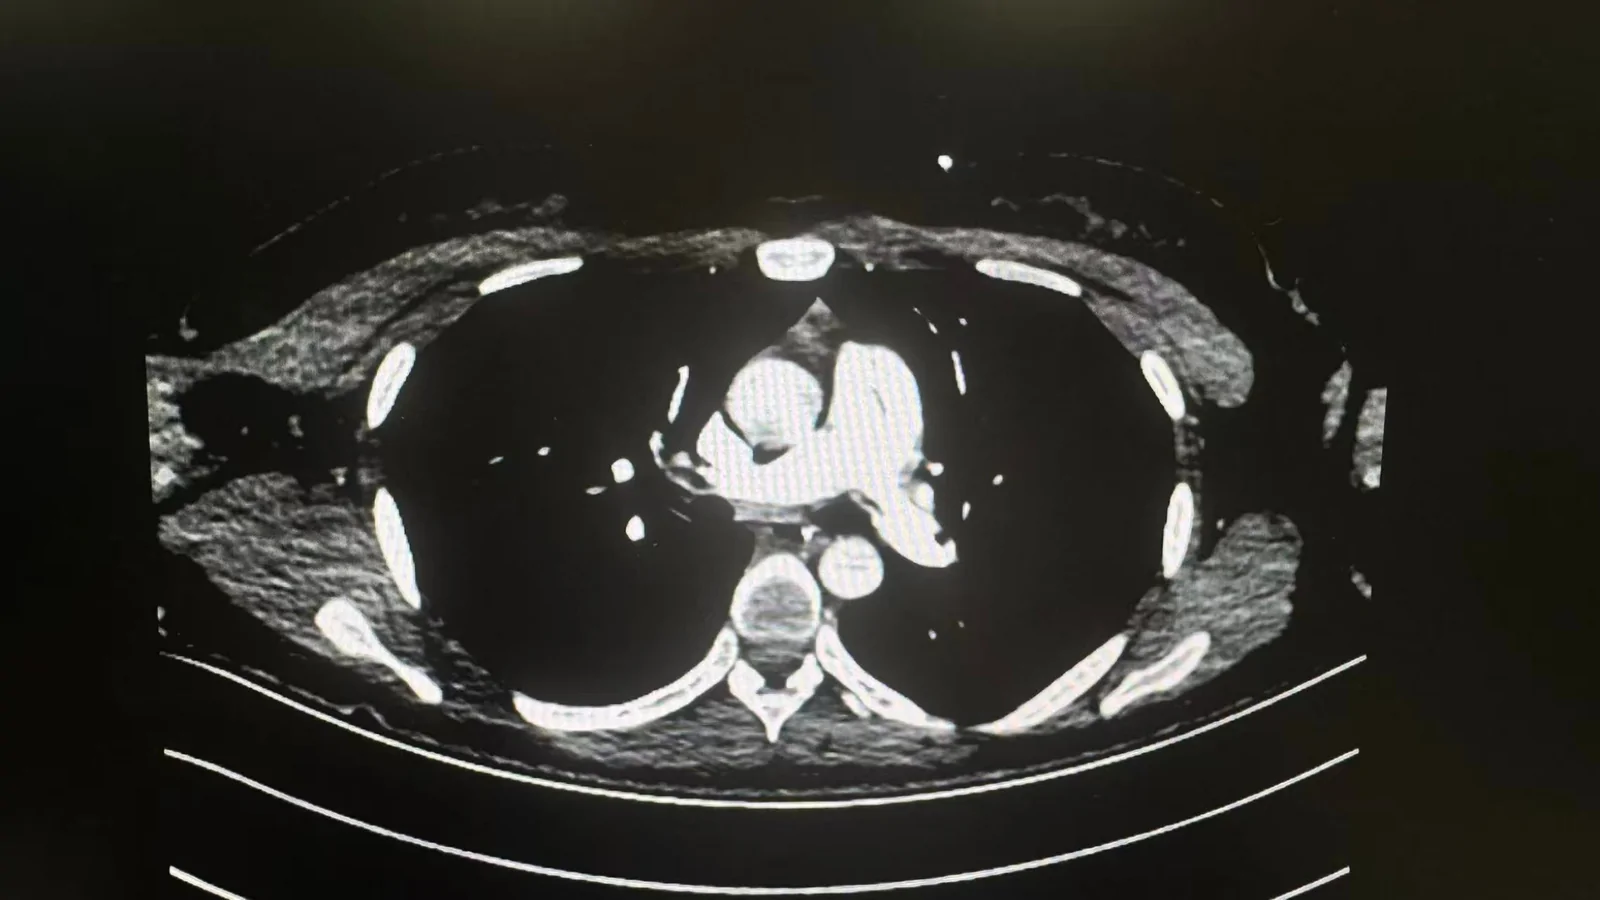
Follow-up CTPA after treatment showing complete resolution of pulmonary arterial thrombus. CTPA = computed tomography pulmonary angiography.
